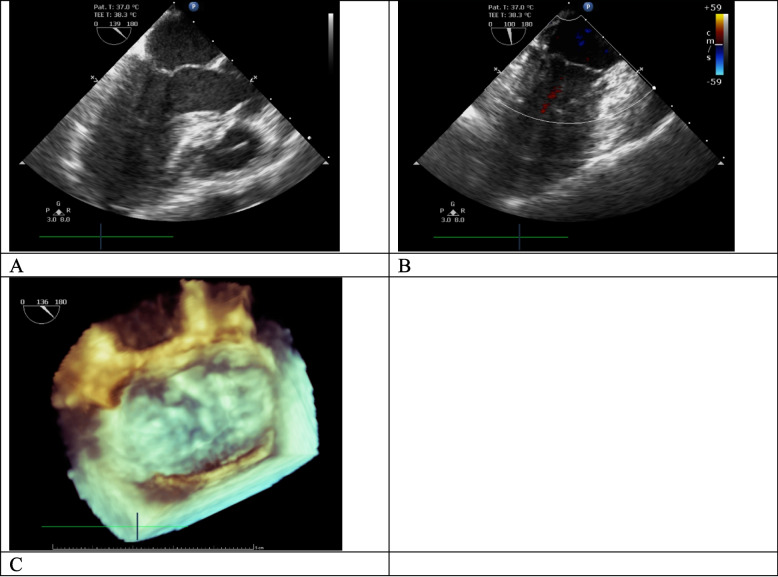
Postoperative echocardiography findings. Postoperative transesophageal echocardiography demonstrated successful mitral valve repair using the autologous pericardial patch (A), with no mitral regurgitation noted (B). The valve leaflets exhibited adequate coaptation during systole (C)
